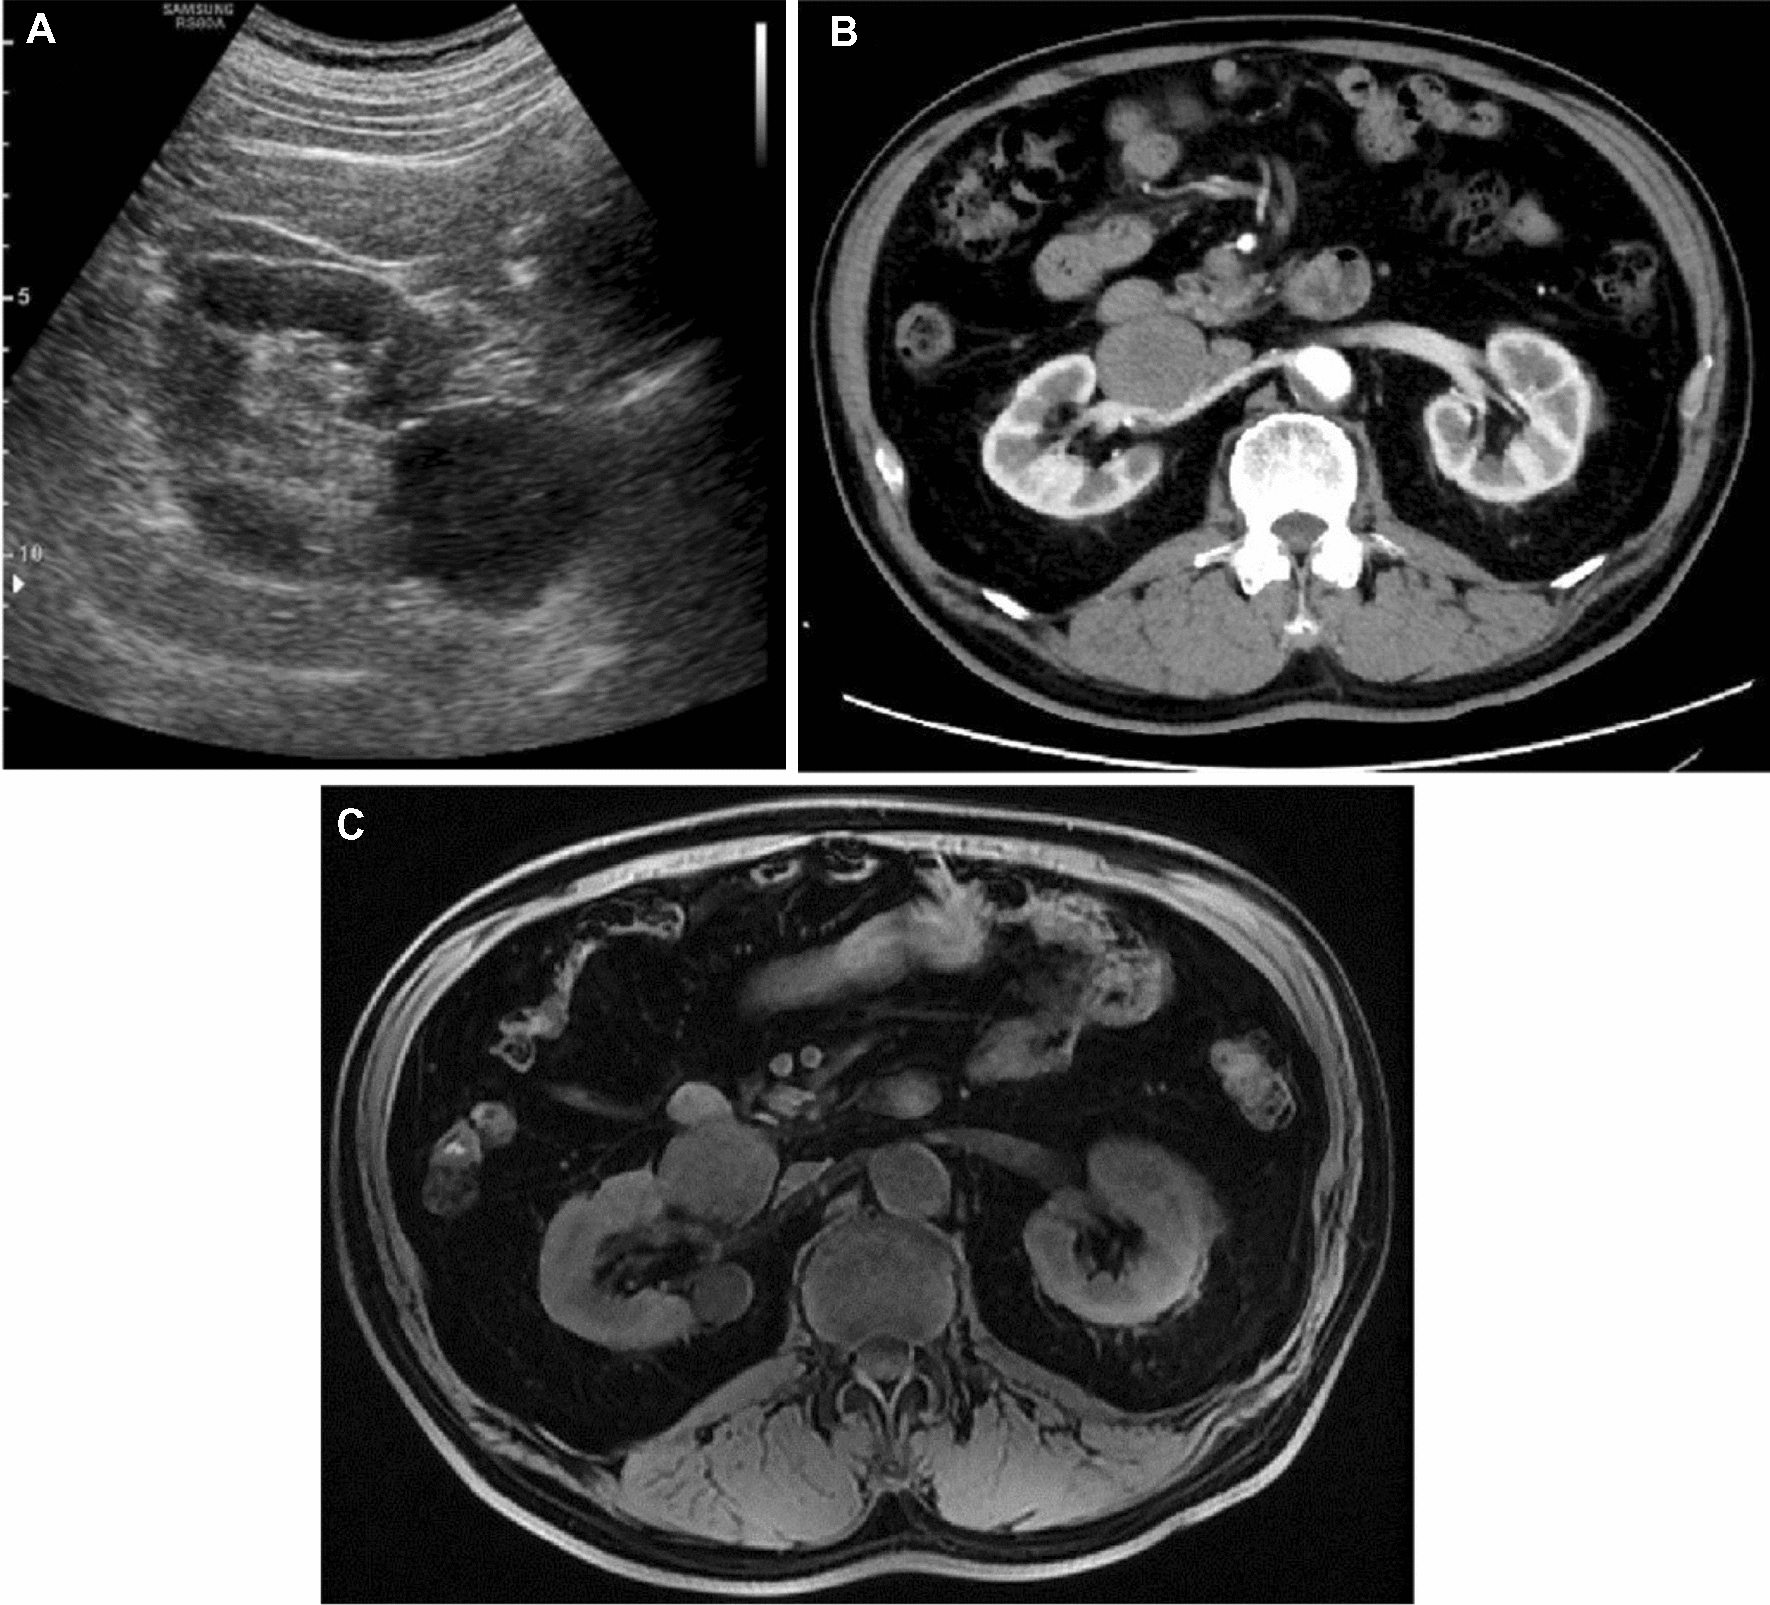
Preoperative abdominal ultrasonography (A), abdominal CT (B), and abdominal MRI (C)
